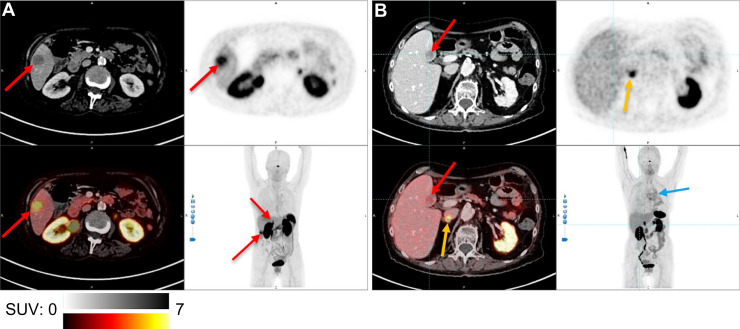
[^68^Ga]Ga-SSO120 PET/CT of liver metastases. Transversal CT (upper left), [^68^Ga]Ga-SSO120 PET (upper right), fused [^68^Ga]Ga-SSO120 PET/CT (lower left), and maximum-intensity-projection (lower right) images shown. (A) Two SCLC liver metastases (red arrows) were detected by [^68^Ga]Ga-SSO120 PET during reinduction therapy with carboplatin plus etoposide. (B) SCLC liver metastasis with no [^68^Ga]Ga-SSO120 uptake 3 mo after end of 4 cycles carboplatin plus etoposide. Primary tumor (blue arrow) was detectable (SUV_max_, 6.2). (A and B) Physiologic uptake in pituitary gland, spleen, liver, adrenal glands (yellow arrows), kidneys, and bladder is also shown.
